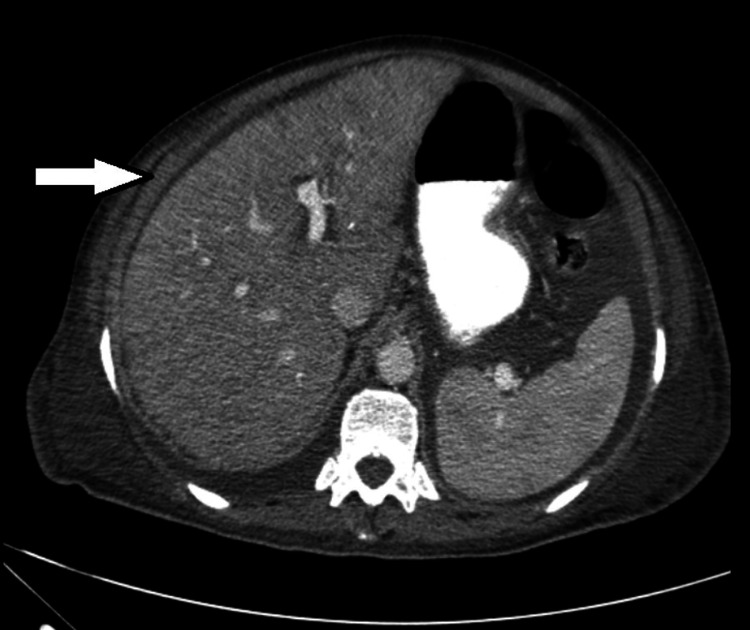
Abdominal CT scan showing hepatomegaly and ascites
